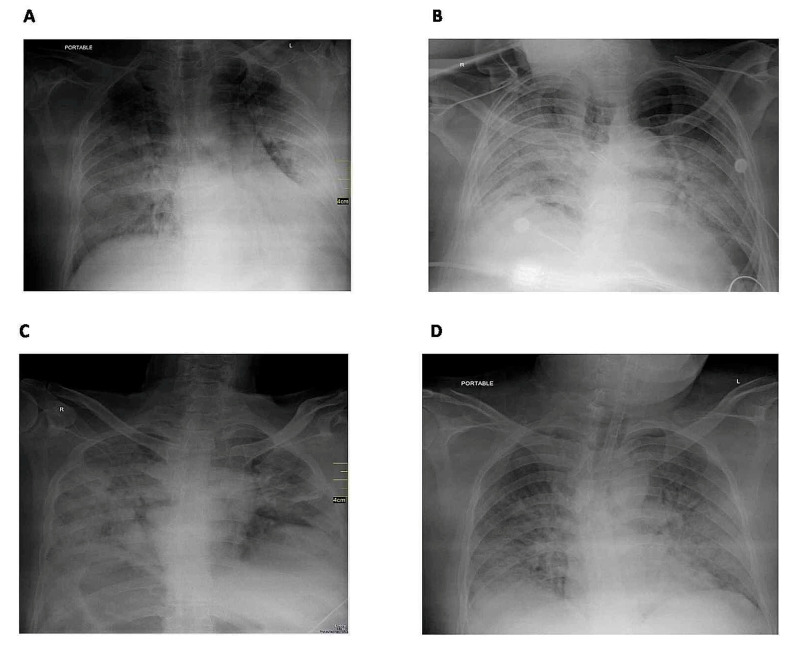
Chest radiographs A: chest radiograph of a 44-year-old male, showing bilateral peripheral, middle, and lower zone patchy consolidation with ground-glass opacities. B: chest radiograph of a 75-year-old male, showing bilateral peripheral, middle, and lower zone patchy consolidation with ground-glass opacities. C: chest radiograph of a 53-year-old male, showing bilateral peripheral, middle, and lower zone diffuse consolidation with ground-glass opacities. D: chest radiograph of a 41-year-old male, showing bilateral peripheral, middle, and lower zone patchy peripheral ground-glass opacities
